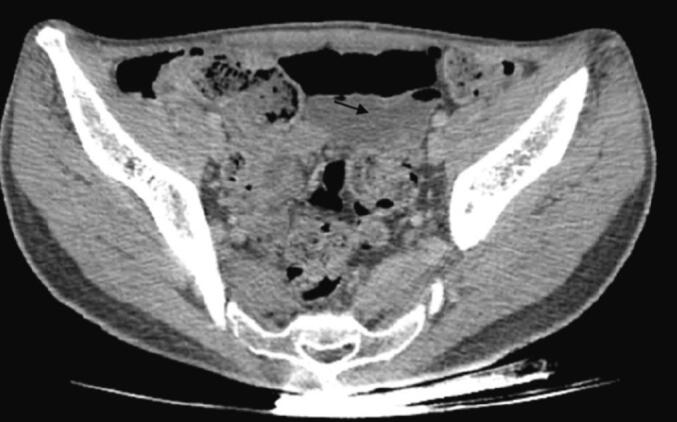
Axial CT section showing pelvic hemoperitoneum.
